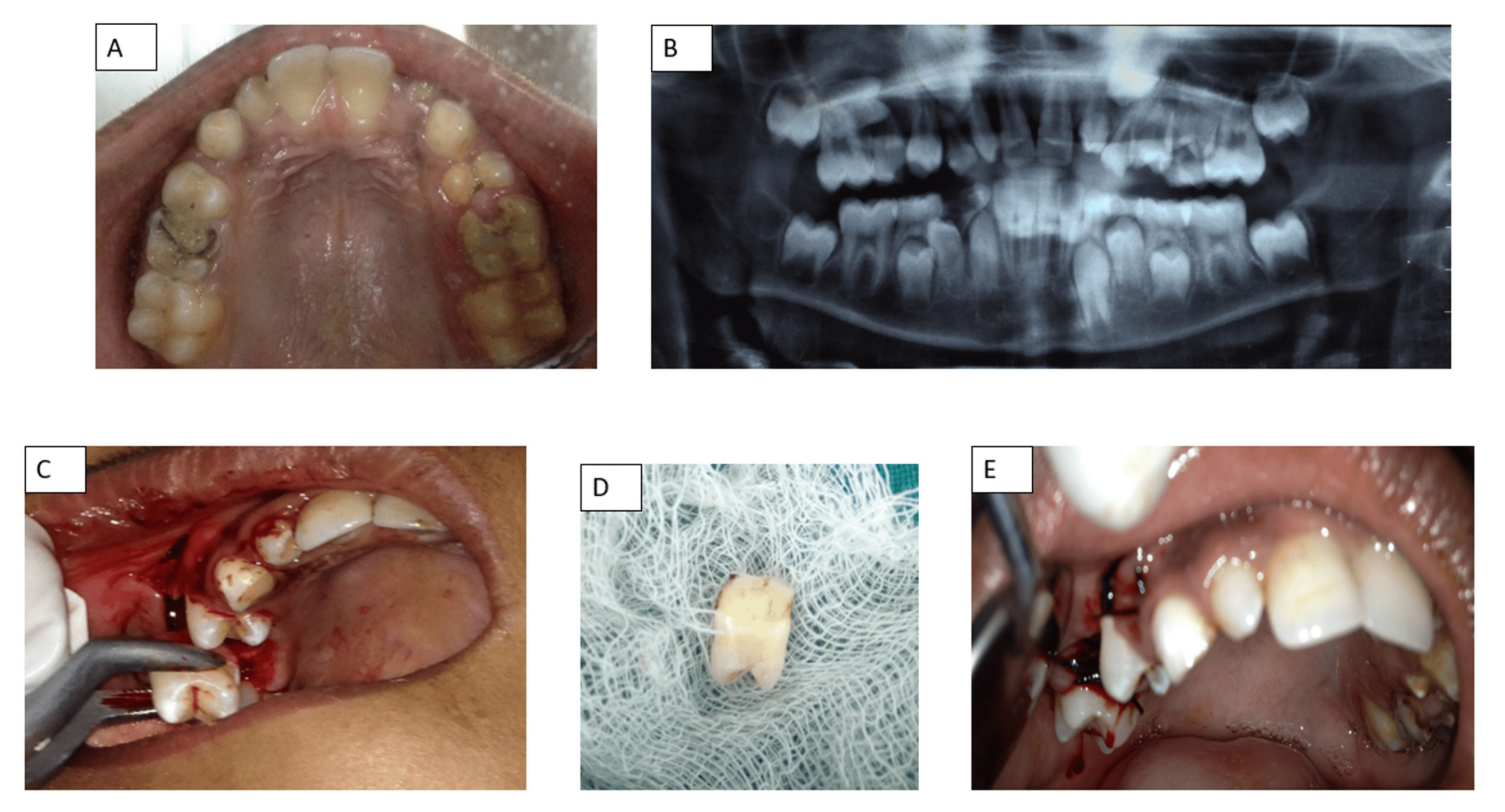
A to E: (A) Preoperative view showing carious 55 and 65; (B) Orthopantomogram showing impacted 15 with unusual transverse long axis and incompletely formed root; (C) Buccal full-thickness mucoperiosteal flap raised; (D) Extracted maxillary right second premolar; (E) Postoperative suturing with 3-0 silk sutures
